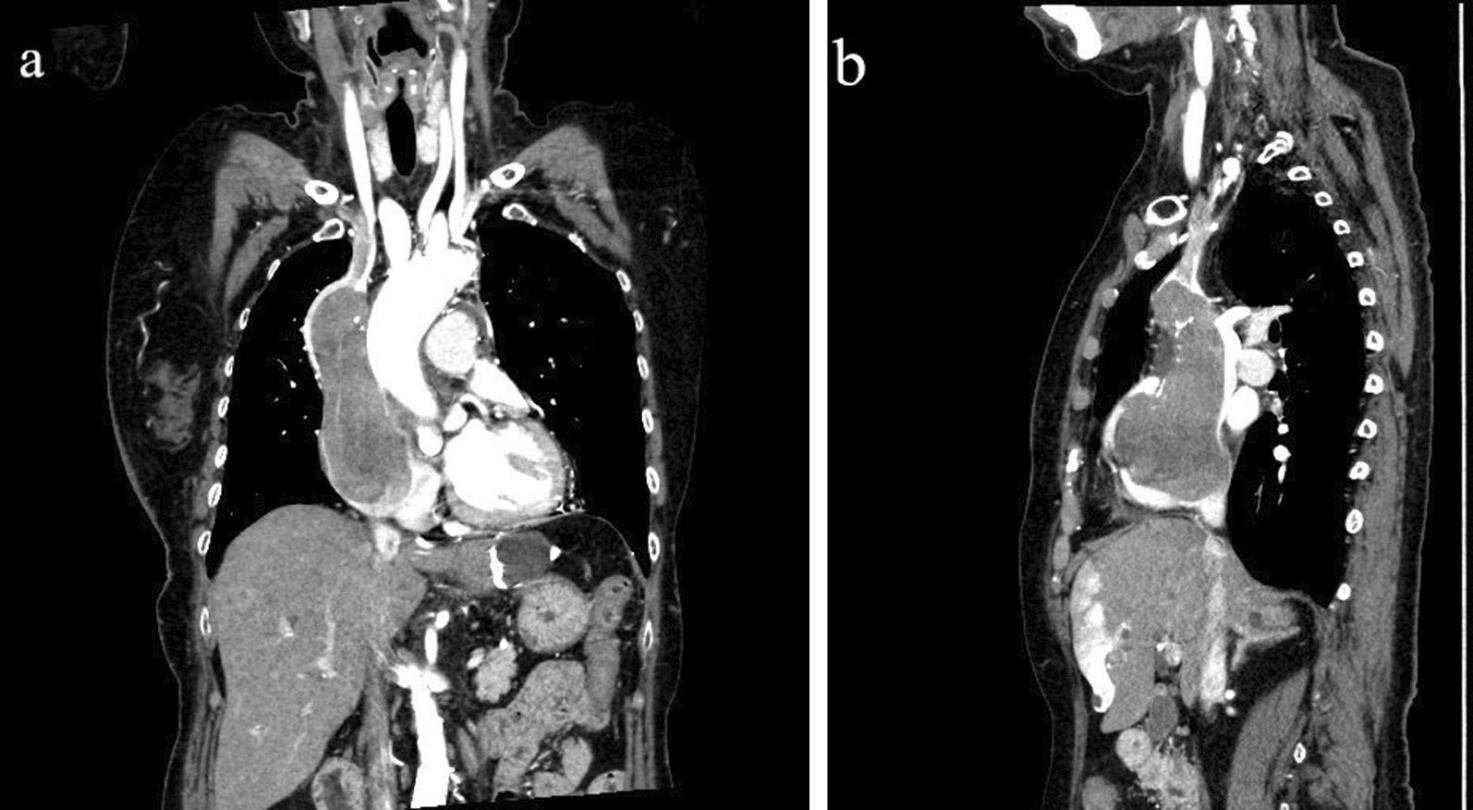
Preoperative contrast-enhanced computed tomography shows a large mass in the right atrium extending to the superior vena cava and left brachiocephalic vein. a Coronal view; b sagittal view
